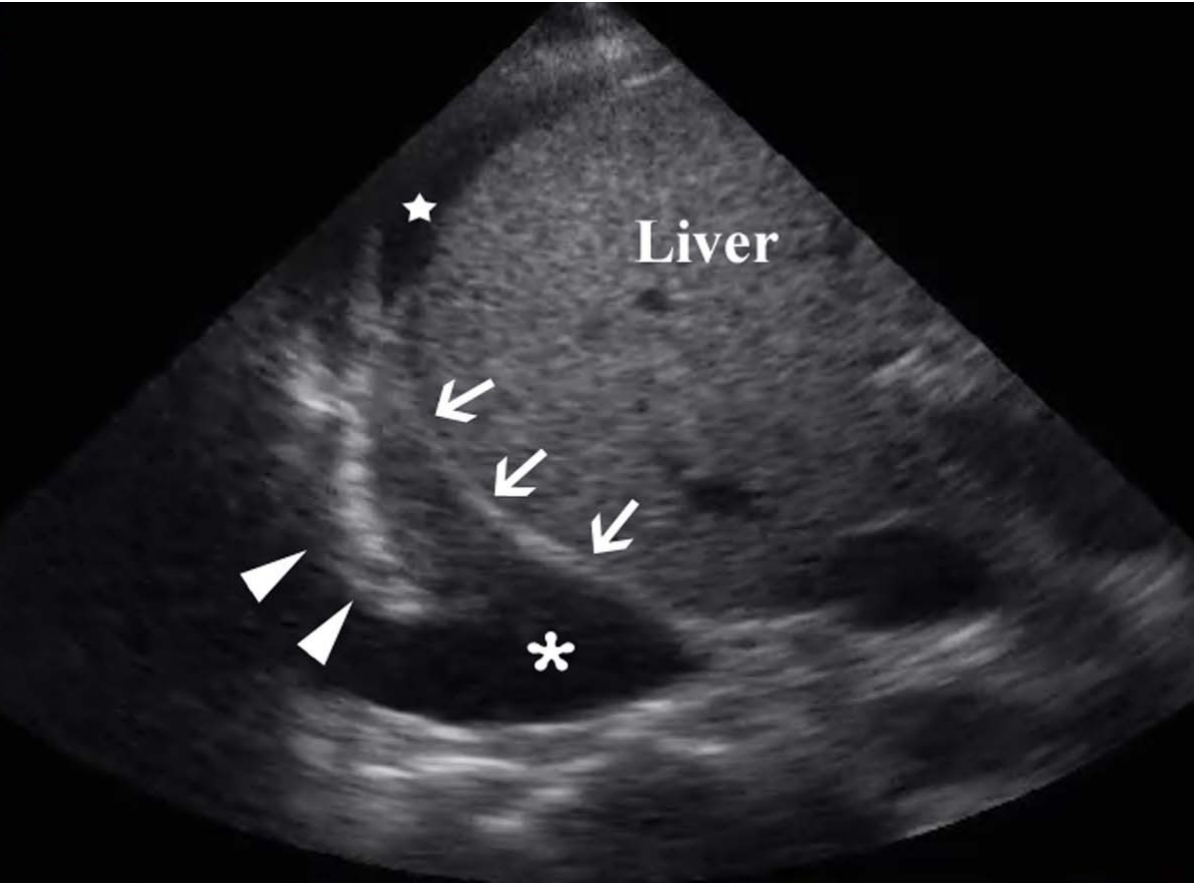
Resuscitative ultrasound image of large pleural collection (*) seen here above the diaphragm (arrows) around the collapsed right lower lobe (arrowheads). Note also the free intra-abdominal fluid (Black star).
